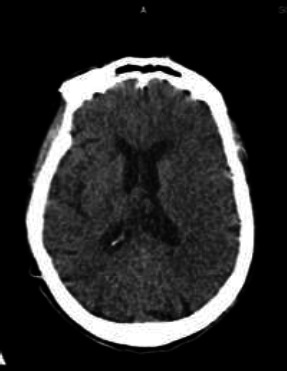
Plain CT brain showing no haemorrhage or acutely demarcated infarct
